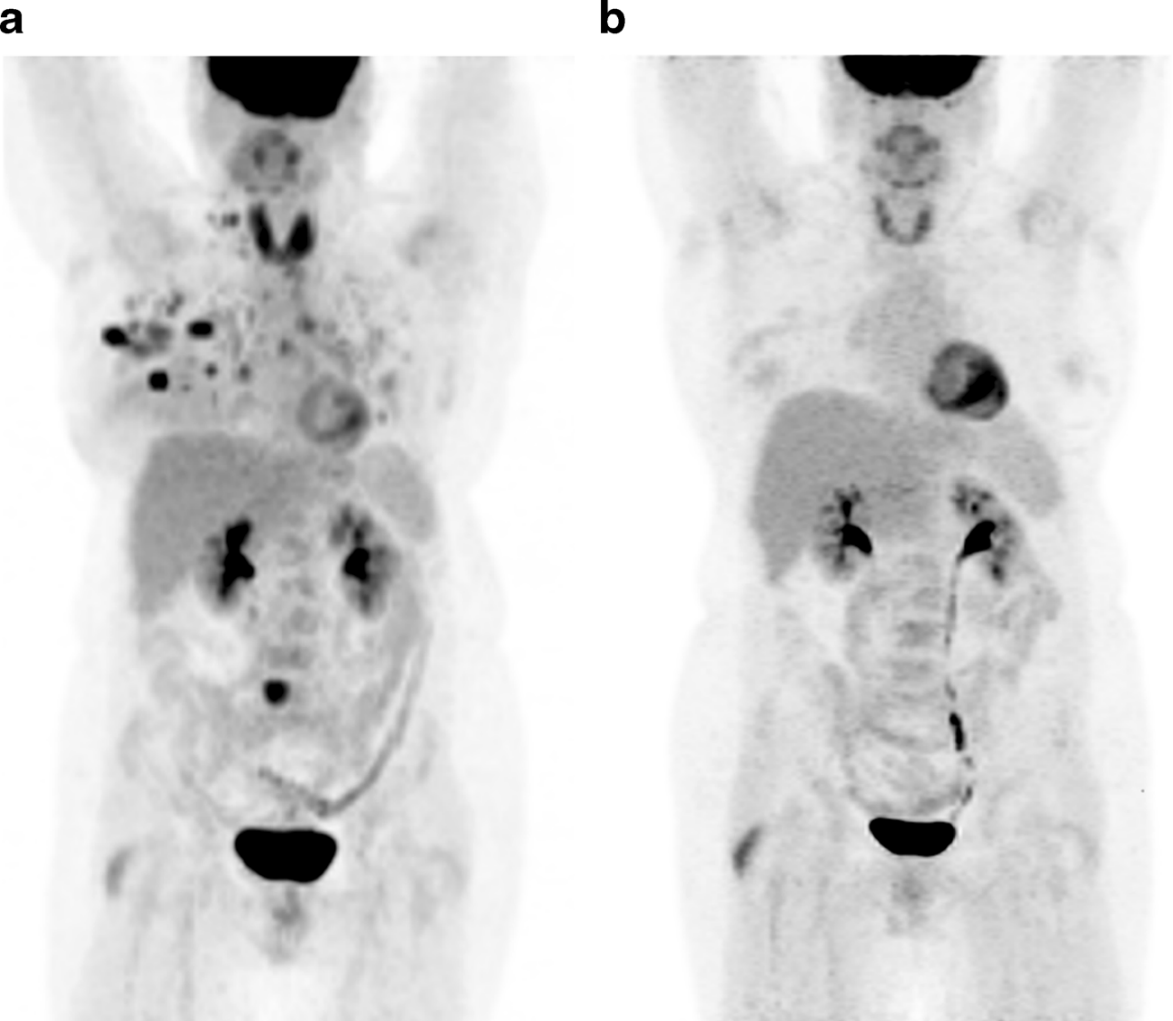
36-year-old woman with invasive lobular carcinoma in the right breast (same patient as in Fig. 4). a Coronal maximum-intensity projection (MIP) 18F-FDG PET image also performed prior to start of treatment shows multiple FDG-avid nodules in the right breast, with right axillary, subpectoral, cervical, mediastinal, and bilateral hilar lymphadenopathy. Also note the FDG-avid focus in the sacrum suggestive of osseous metastasis. b Coronal maximum-intensity projection (MIP) 18F-FDG PET image after 6 months of therapy shows significant decrease in FDG-avid lesions suggestive of response to therapy
